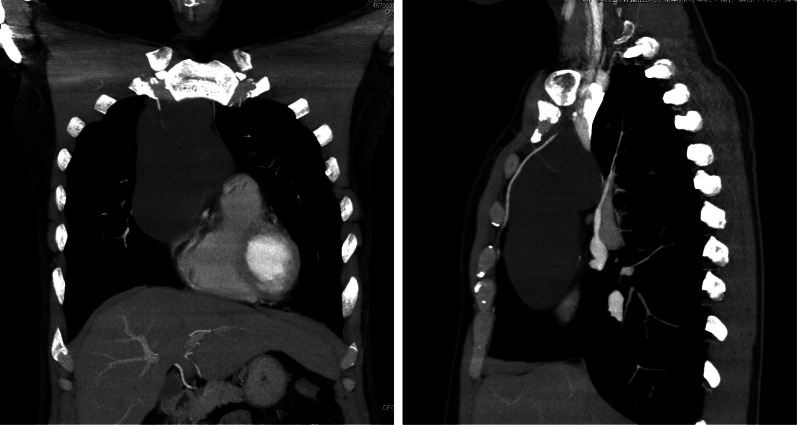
Enhanced CT revealed a lobulated homogenous giant mass in the superior-to-anterior mediastinum. The components of the cyst had increased CT attenuation of 10 HU, which is higher than that of water
